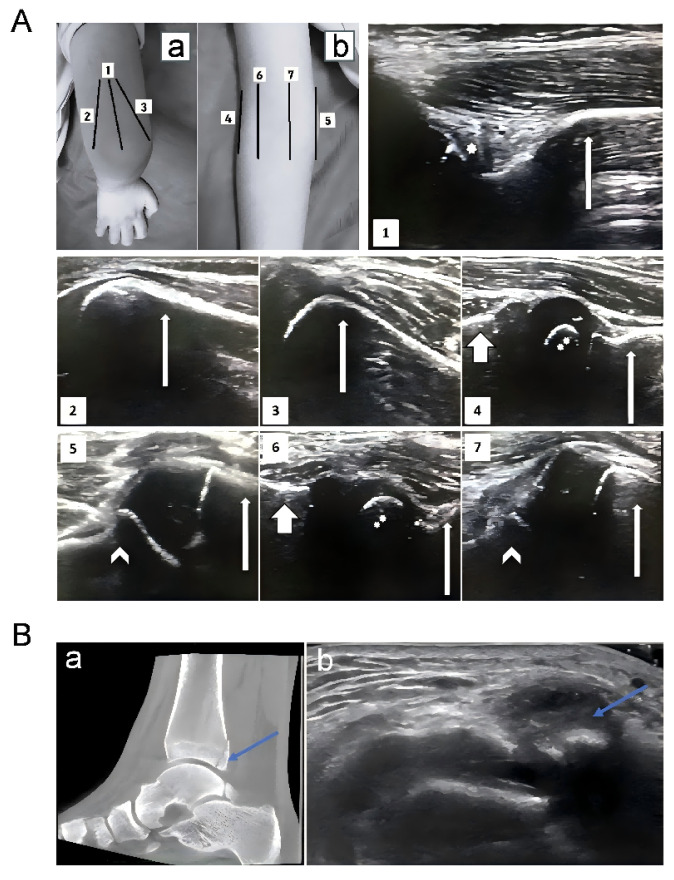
(A): (a): Elbow flexion position and posterior of humerus; (b): Elbow extension position anterior of elbow, (1): Dorsomedian aspect, (2): Dorsoulnar aspect, (3): Dorsoradial aspect, (4): Lateral aspect, (5): Medial aspect, (6): Ventroradial aspect, (7): Ventroulnar aspect, asterisk: posterior fat pad, double asterisks: Capitellum, long arrow: Humerus, Short arrow: Radius, arrowhead: Ulna [137]. (B): A fracture of the posterior malleolus of the tibia seen on CT (a) and ultrasound with a deltoid ligament rupture (b) in the same patient (arrow) [138].
